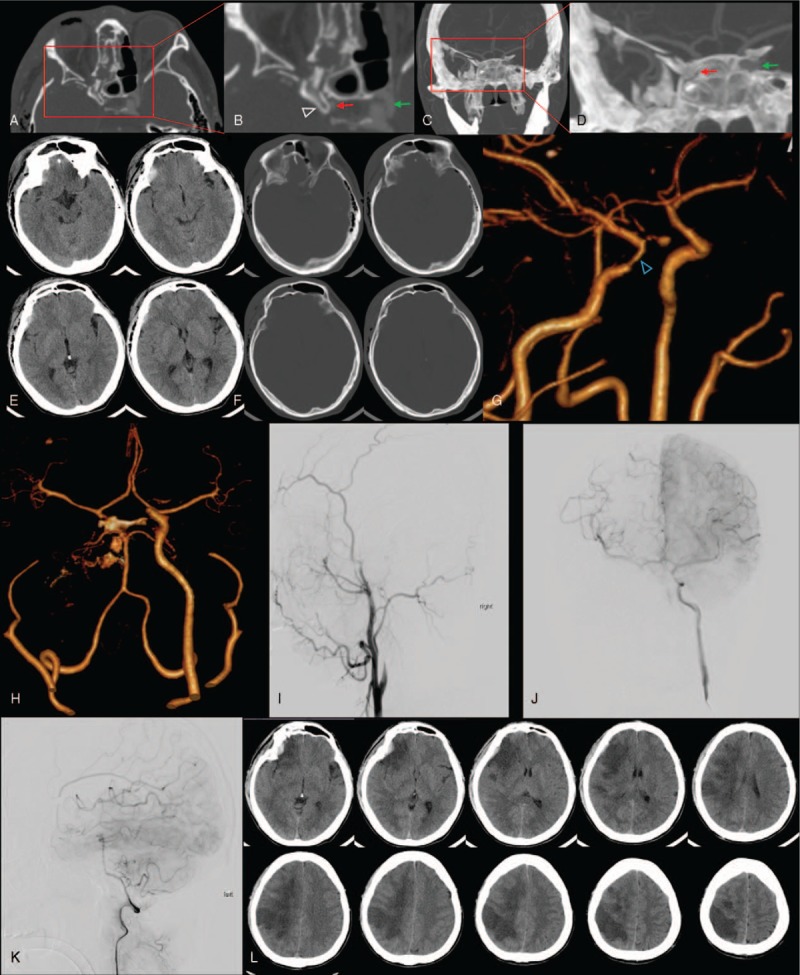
Figures from case 1. (A) The orbit computed tomography (CT) and (B) the enlarged figure from red square. The white hollow arrow indicates anterior clinoid process (ACP), red arrow indicates right internal carotid artery (ICA), and green arrow indicates left ICA. The right ICA is extruded by ACP. (C) The coronal scan and the (D) enlarged figure from rad square. (E) and (F) The initial head CT scan. (G) The 3D reconstructed image of head CT angiography (CTA). The blue hollow arrow refers to arterial stenosis. (H) The second CTA. The right ICA disappeared. The digital subtraction angiography (DSA) figures of right ICA (I), left ICA (J), and left vertebral artery (K). (L) The head CT scan showed cerebral infarction.
